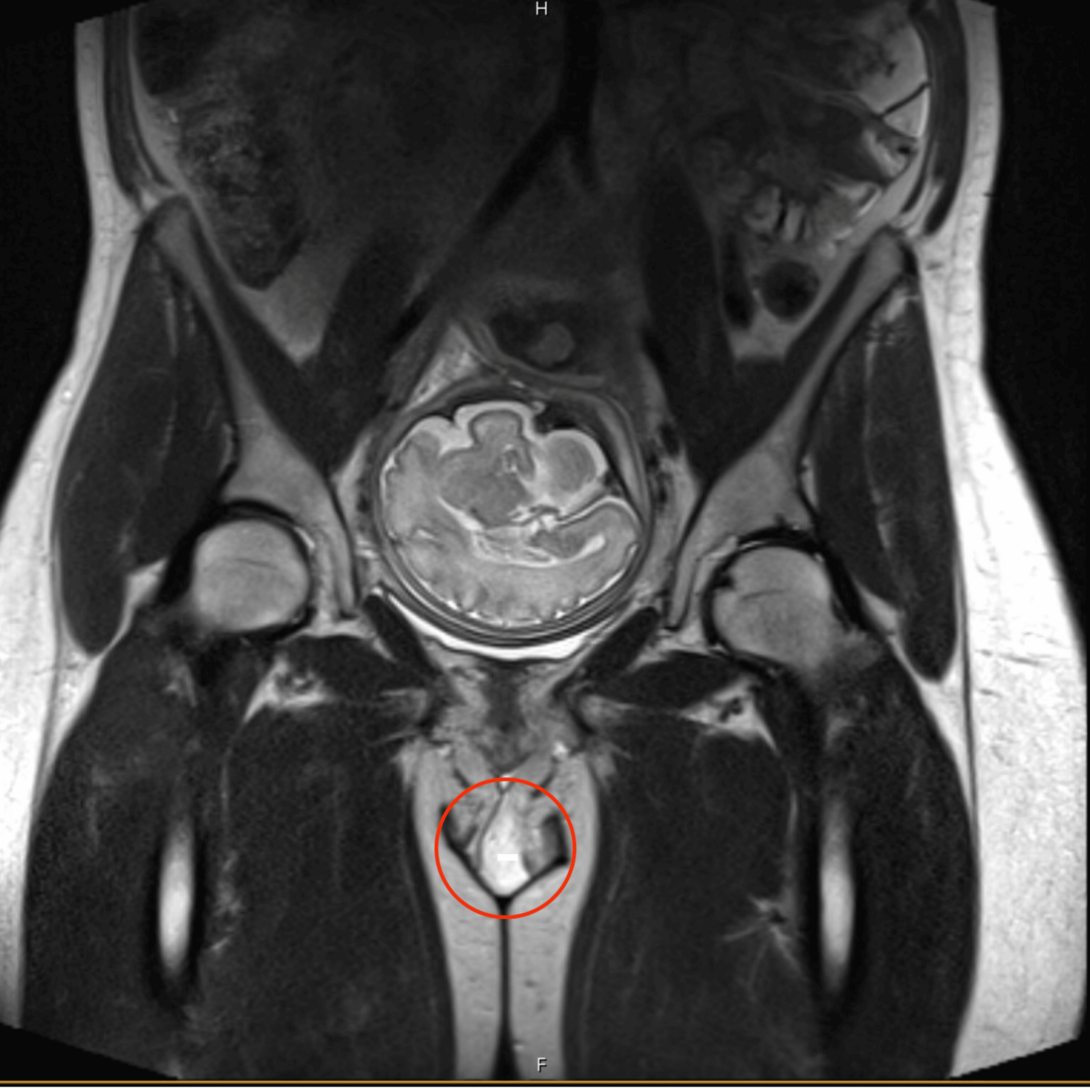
MRI of the pelvis without contrast Coronal view showing edematous labia. The image also demonstrates cephalic presentation of the fetus.
